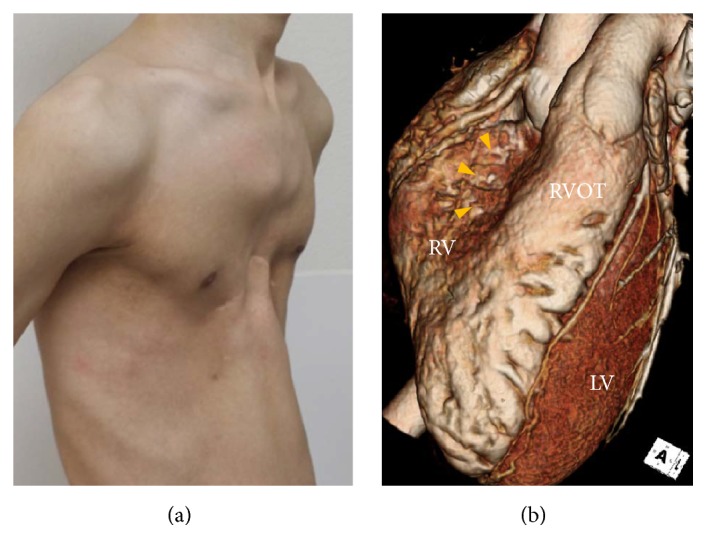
(a) Gross picture shows a deformity of the anterior thoracic cage consistent with pectus excavatum. (b) 3D reconstructed computed tomography image also reveals external compression of basal-to-mid portion of the right ventricle (yellow arrow heads). LV, left ventricle; RV, right ventricle; RVOT, right ventricular outflow tract.
